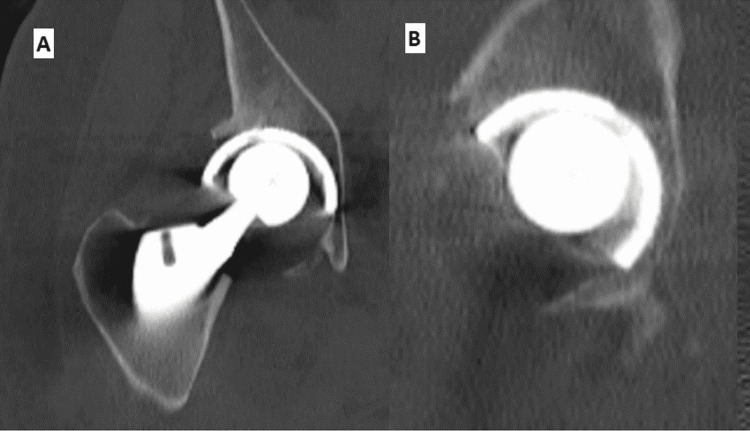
CT scan showing PLD (A) Coronal view and (B) sagittal view
